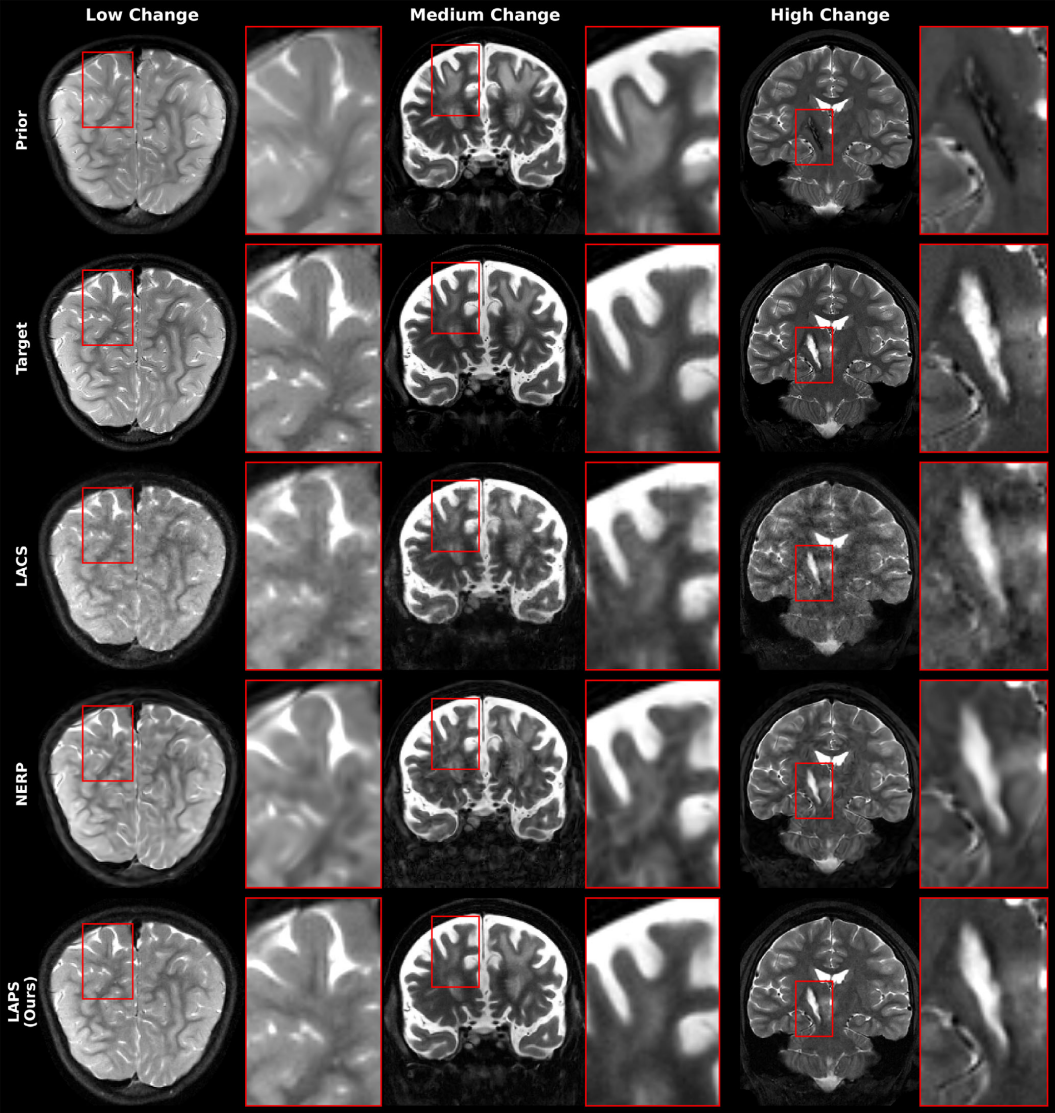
Comparison of different longitudinal reconstruction algorithms across varying degrees of change between scans, for a 2D undersampling case with R=20. The first row shows the prior scan, the second row shows the new scan (target), and the subsequent rows present reconstructions from different methods, with our proposed method (LAPS) shown in the last row. Left column: Axial $T_{2}$‐weighted slice from the same patient shown in Figure 3, now displaying a region with minimal or no change between scans. Middle column: Coronal $T_{2}$‐weighted images of a patient with a brainstem tumor (not shown). The displayed slice shows $T_{2}$‐hyperintense white matter changes related to treatment, along with white matter swelling that decreases on the follow‐up MRI performed 6 days later. Right column: Coronal $T_{2}$‐weighted images of a brain with an involuted right basal ganglia hematoma, secondary to a ruptured arteriovenous malformation. The initial exam reveals a $T_{2}$‐hypointense contracted cavity corresponding to old blood products. The follow‐up exam, performed 4 months later, shows fluid accumulation within the cavity and $T_{2}$‐hyperintense edema in the surrounding brain parenchyma due to interval radiosurgery treatment. The automatically selected projection times $t_{p}$, estimated by AutoInit, were 280, 332, and 453 for the left, middle, and right columns, respectively, reflecting increasing levels of change across the three cases.
